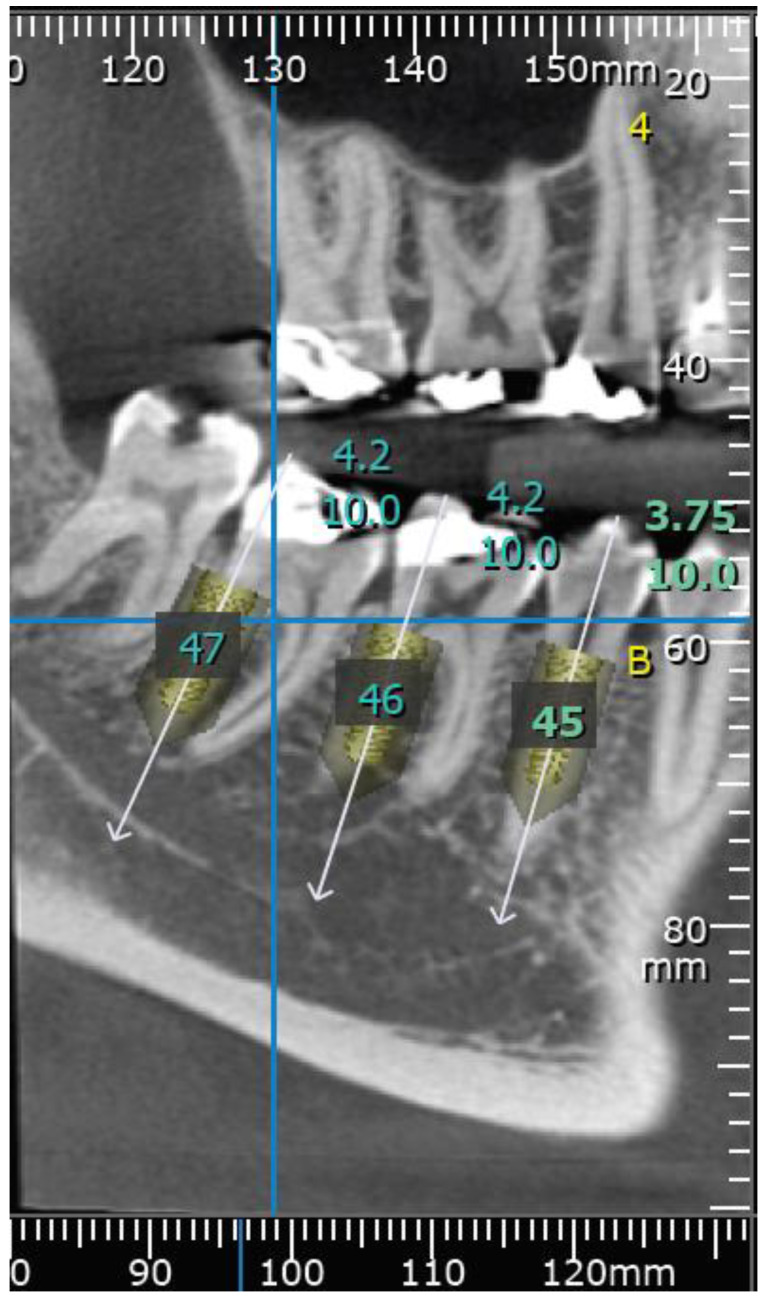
Virtual implant placement of 3 dental implants at right posterior mandibular sites: second premolar (3.75 mm diameter and 10mm length), distal root of first molar, and distal root of second molar (4.2 mm diameter and 10mm length each). CBCT para-axial sagittal section.
